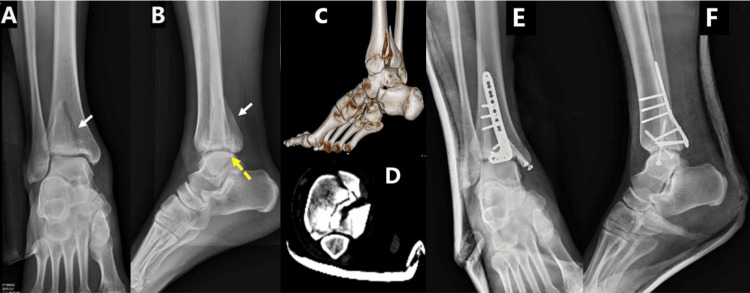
Surgical fixation of a Haraguchi type 2 posterior malleolar fracture using a plate A 58-year-old male sustained a fall from a height of 2 meters, resulting in a right ankle fracture involving the posterior malleolus. Preoperative radiographs (A and B) showed the fracture, with white arrows indicating the affected area. Although the lateral view (B, yellow dotted arrow) suggested minimal involvement of the articular surface, a CT scan (C, D) revealed medial extension of the fracture, classifying it as Haraguchi type 2. To address this, a posteromedial approach with plate fixation was chosen for internal fixation. Postoperative imaging confirmed the successful restoration of the articular surface (F). A, B: Preoperative AP and lateral radiographic views; C: 3D reconstruction based on a CT scan; D: Transverse section of the CT scan at the plafond level; E, F: Postoperative AP and lateral views
